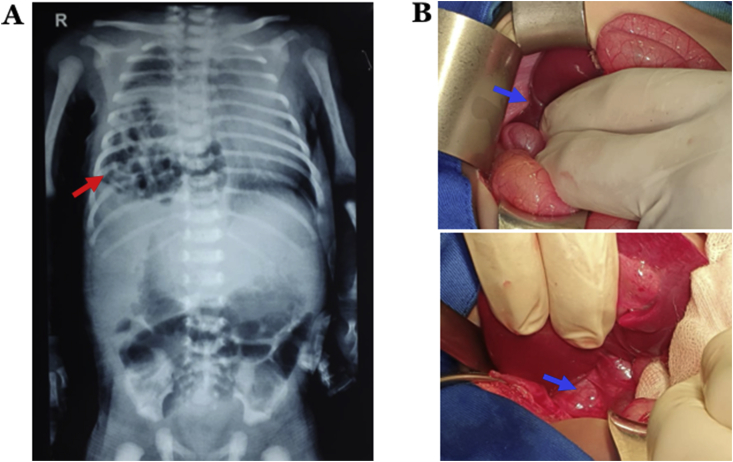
A) Chest X-ray showed intestinal gases on the right diaphragm and elevation of the right diaphragm. B) Intraoperative findings revealed elevation of the right diaphragmatic dome and visceral displacement, including the ileum, transverse colon, and right lobe of liver; and no diaphragmatic rupture was found.
